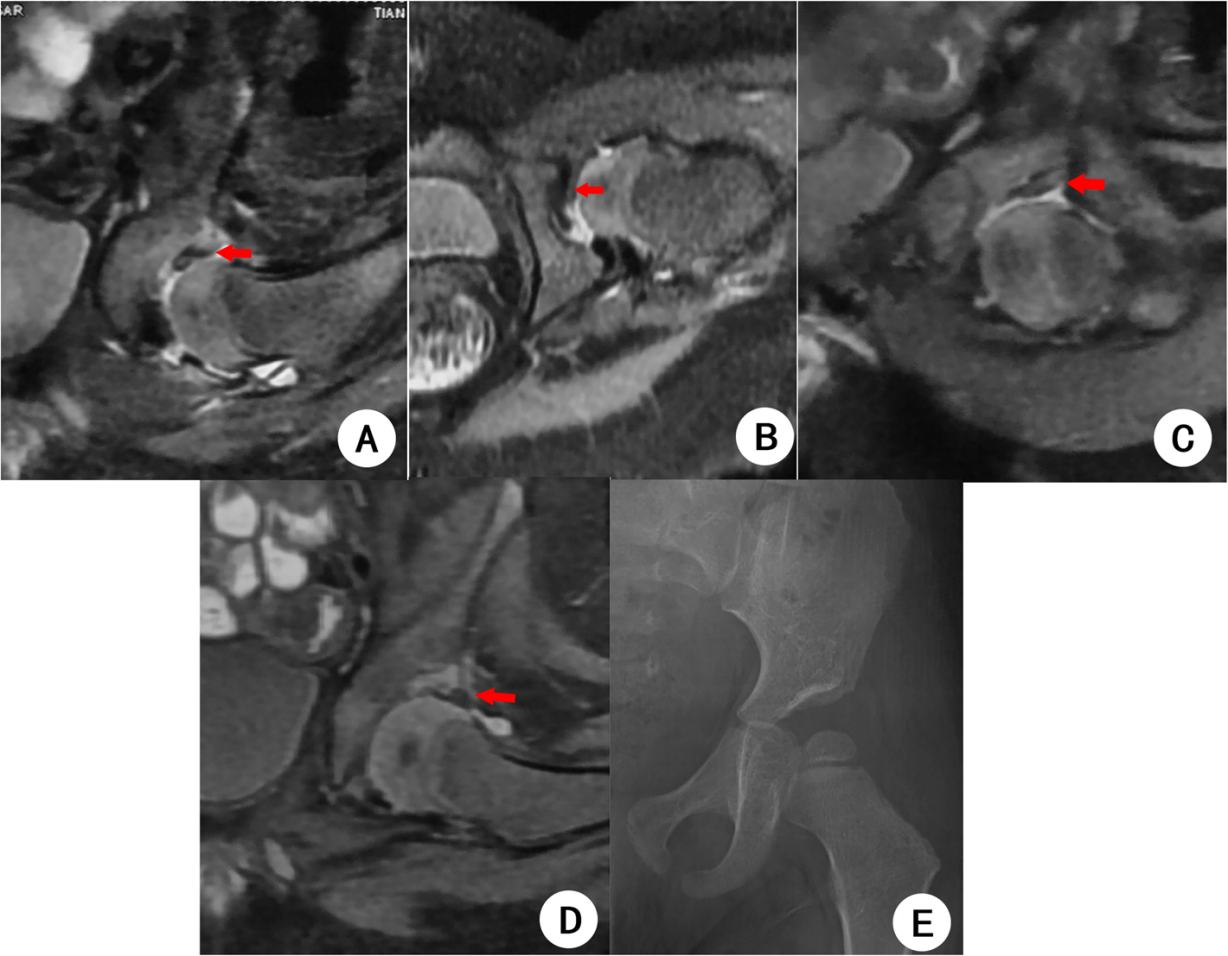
A female patient 10 months after closed reduction and spica casting of the left hip joint. The patient underwent MRI examination of both hips immediately after CR, 3 months after CR, and five and a half months after reduction. a and b show the coronal and transverse FS PDWI images of MRI immediately after CR. It is suggested that the LCC of the left hip is hypertrophied and completely inverted immediately after reduction, which is considered as type 4; the ligamentum teres, transverse ligament, and pulvinar are hypertrophic. c shows that the LCC became flattened 3 months after reduction, and the ligamentum teres, transverse ligament, and pulvinar were less hypertrophic than before. d shows that five and a half months after reduction, the LCC still had partial inversion which was considered as a type 3 complex. The ligamentum teres, transverse ligament, and pulvinar were not thickened and returned to normal, and the left hip joint achieved complete relocation. e shows the pelvic X-ray film of the patient 22 months after closed reduction, the left osseous acetabular index is 33.3°, indicating that there is still residual acetabular dysplasia; the shape of the left femoral head is intact, and no obvious abnormal density is found
